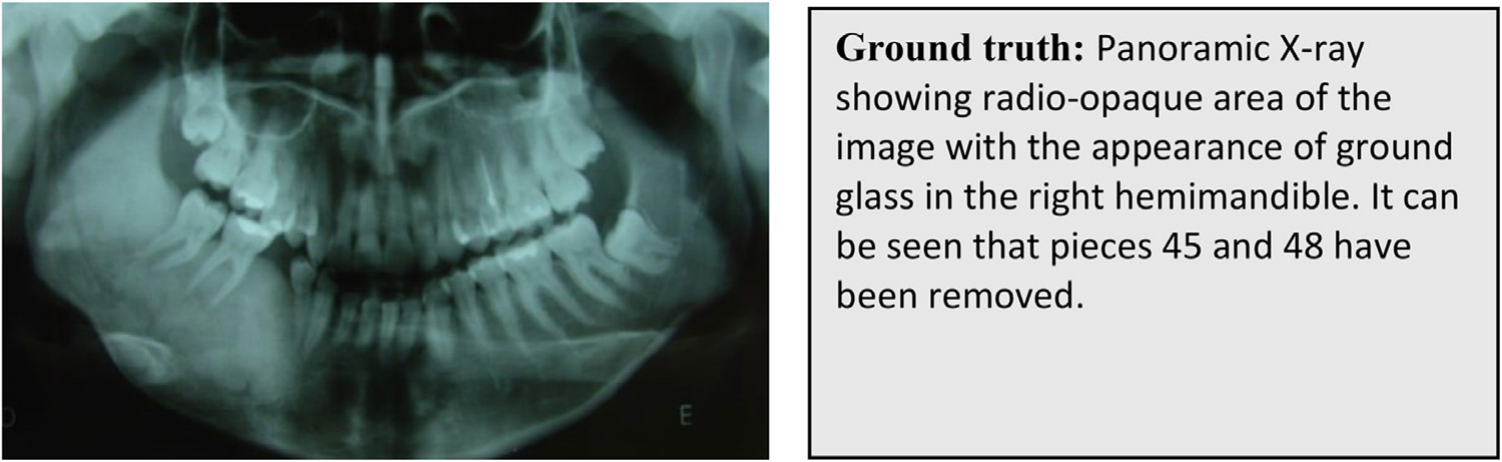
Example panoramic X-ray with corresponding generated reports.
